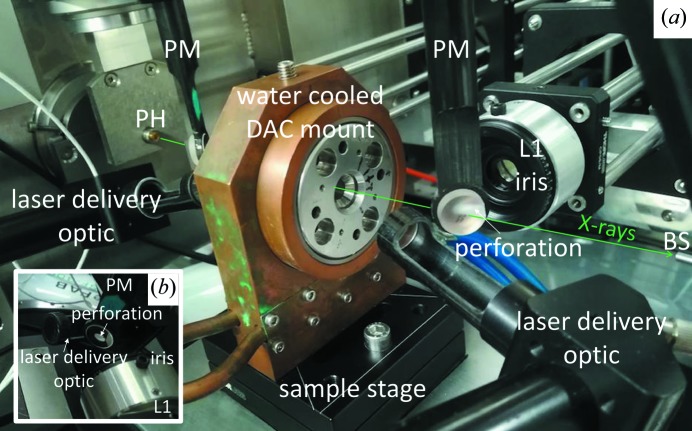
(*a*) Close-up view of the region around the pressure cell (DAC) for the off-axis laser delivery. Both laser delivery optics are visible. Perforated mirrors (PM) in the X-ray path allow the direct X-ray beam to pass through but reflect the thermal emission from the sample towards the collecting optics (only L1 visible). For spatial stability the DAC is water cooled. BS: beamstop. PH: clean-up disk. In the present picture, the iris mounted on L1 is visible. (*b*) Detailed view of the region near a perforated mirror.
